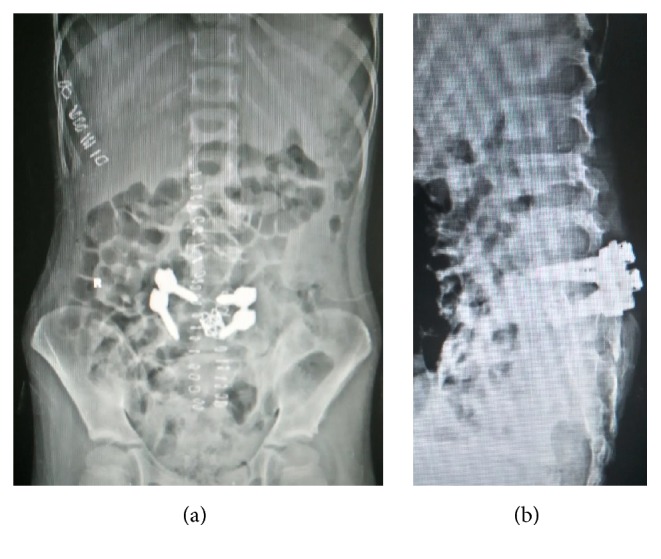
A-P and lateral radiograph of lumbosacral spine following 2nd-stage surgery.
